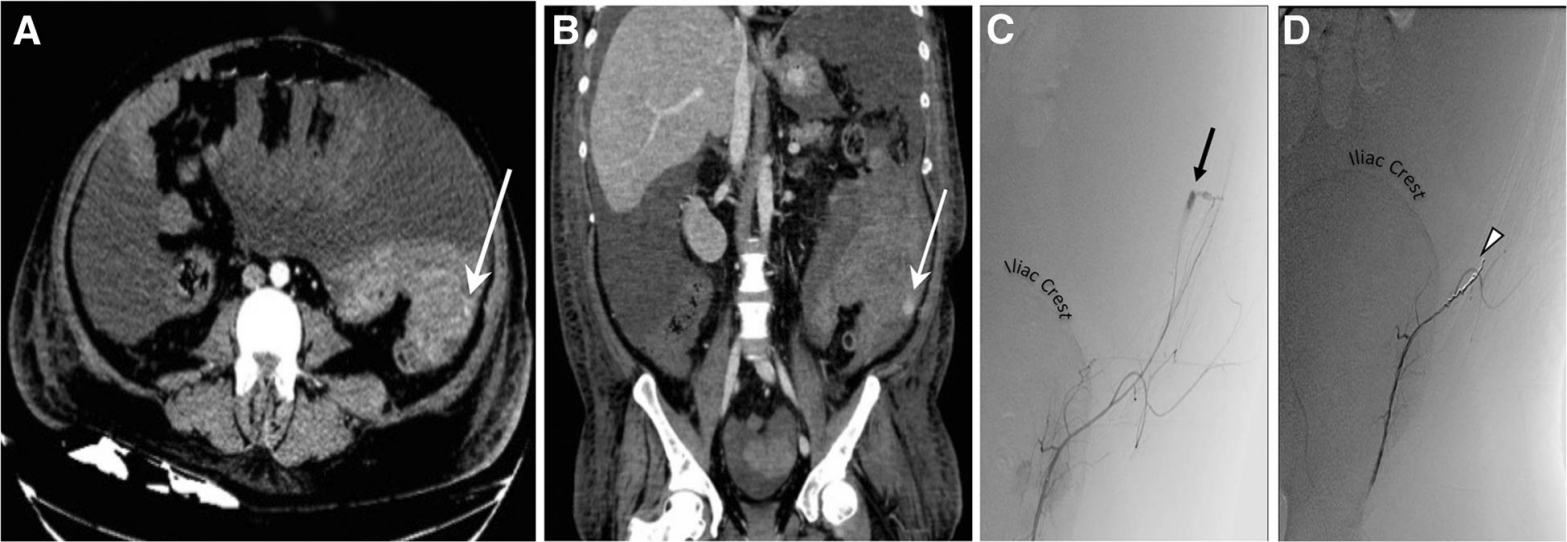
a Axial and b coronal CT images demonstrate ascites with fluid-fluid level suggestive of blood products with active contrast extravasation along the left lateral abdomen (arrows). Subsequent digital subtraction angiography images show extravasation c from an injury to a branch of the left deep circumflex iliac artery (arrow), and d the same patient after coil (arrowhead) and Gelfoam embolization of the vessel indicating successful occlusion of the artery and resolution of hemorrhage
